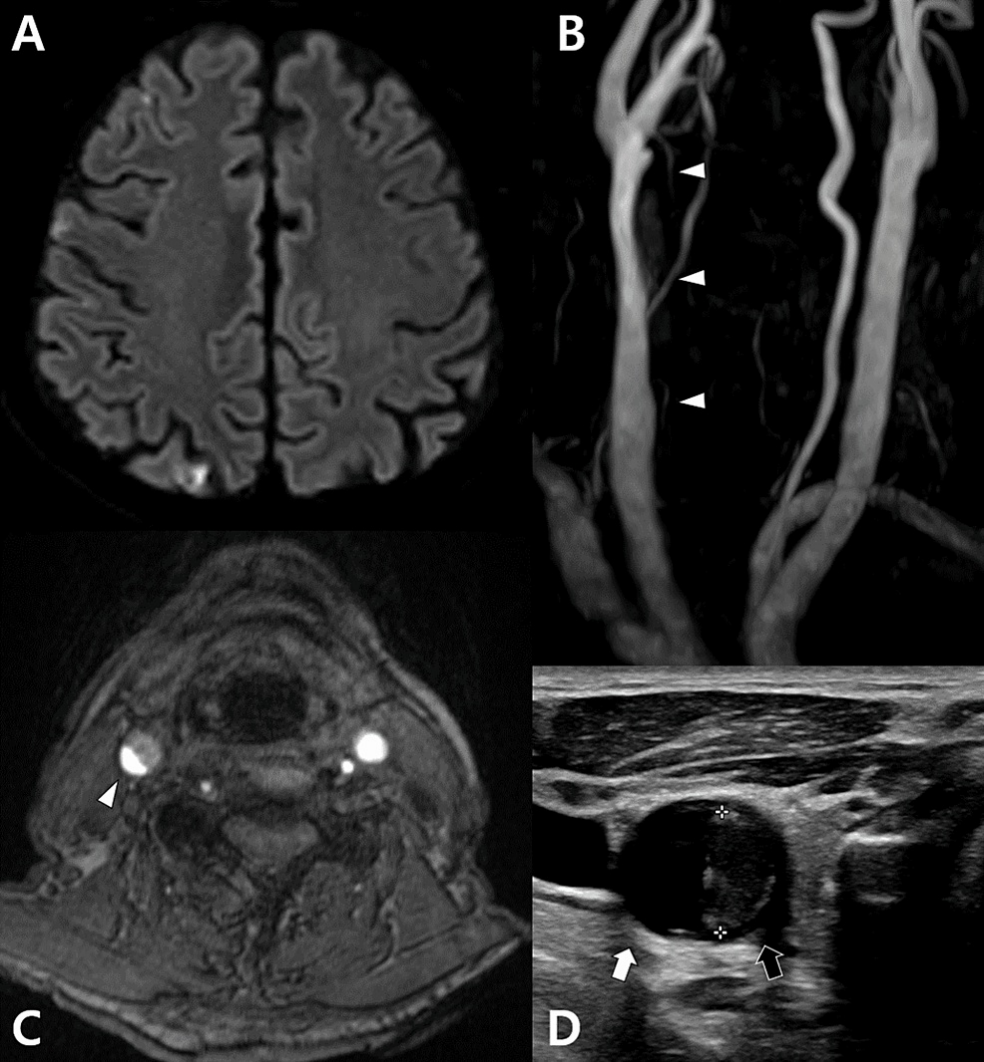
Initial radiological findings (A) Diffusion-weighted magnetic resonance imaging reveals multi-focal infarctions on the right hemisphere. (B, C) Neck magnetic resonance angiogram and its source image show a moderate stenosis (white arrowheads) from the right mid to distal common carotid artery. (D). The cross section of carotid ultrasonography shows a lumen (white arrow) in a semilunar shape and thrombus (black arrow) in the remaining semilunar portion.
